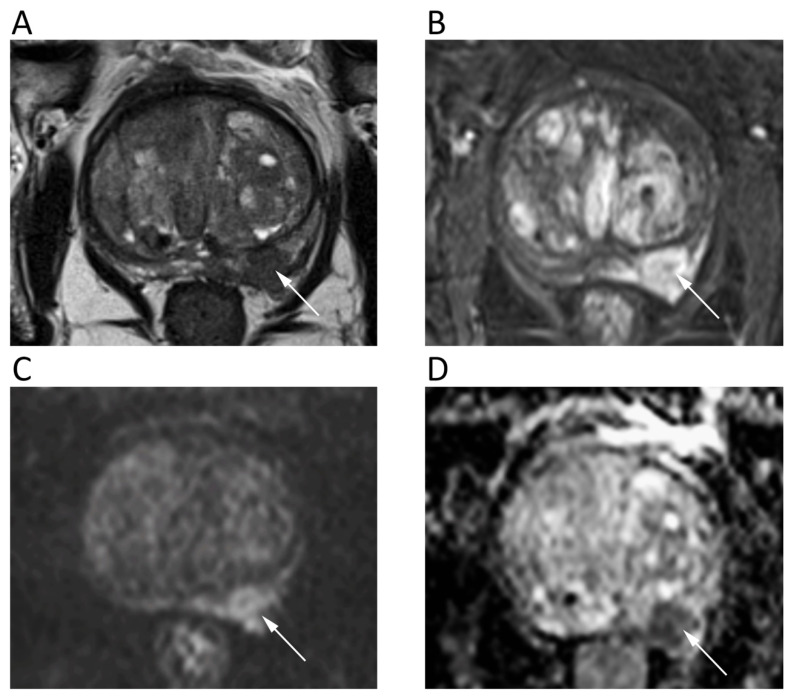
A 68-year-old male patient with prostate cancer. Axial T2w (A) and post-contrast fat-suppressed T1w (B) images show a hypointense nodule in the left peripheral zone (arrows) with high signal intensity on *b* = 1000 DWI image (C) and restricted pattern of diffusion on the corresponding ADC map (D).
